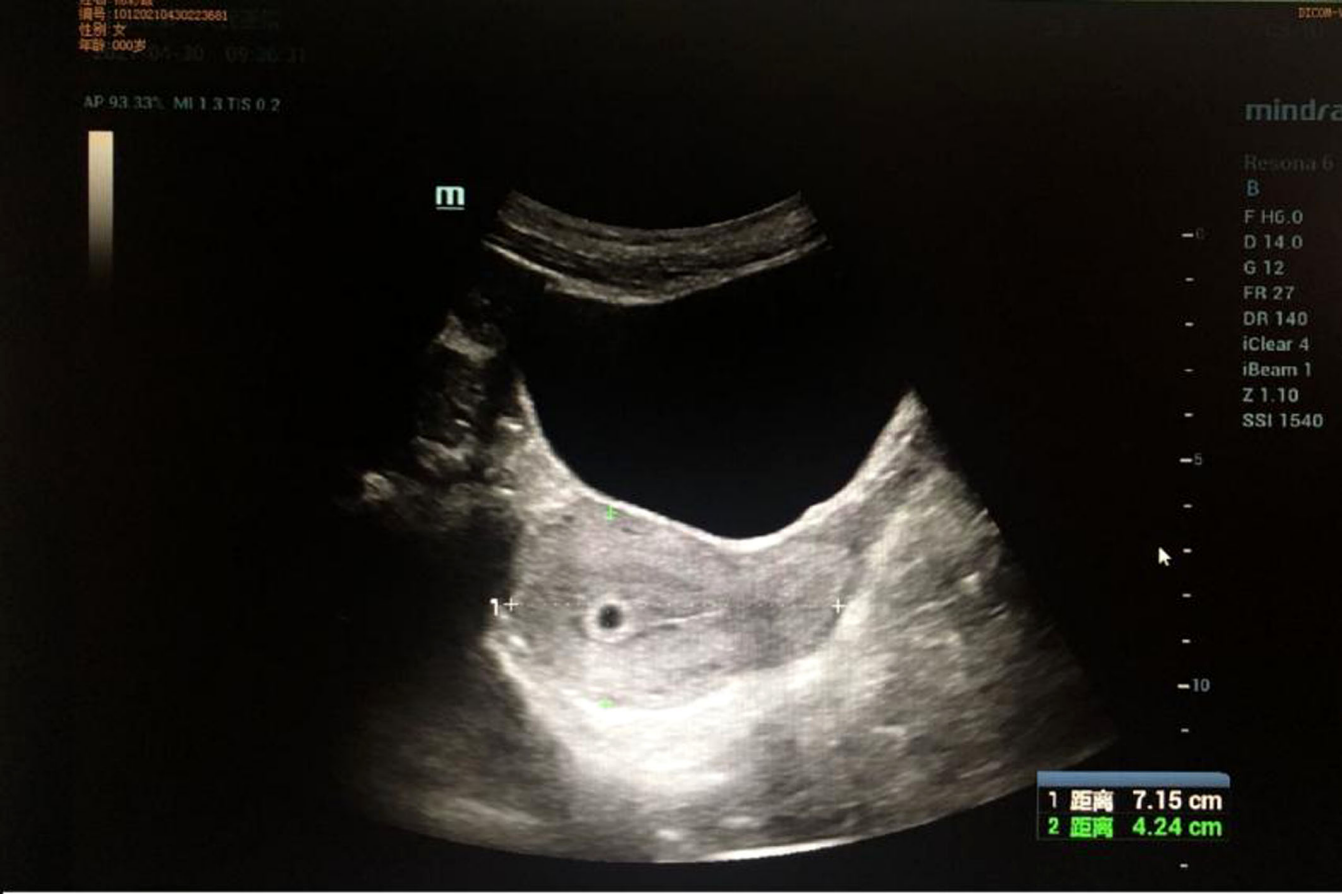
Ultrasound imaging: The gestational sac in the uterus was detected by ultrasound examination.
